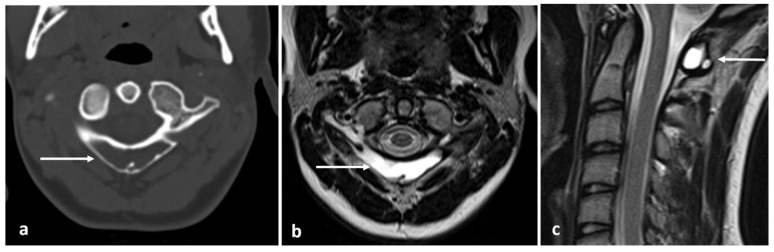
Axial CT (a), T2 (b) and sagittal T2 (c) images of a simple bone cyst of the C1 vertebra (white arrows). Note the lytic appearance on CT and fluid signal on T2 sequences.
